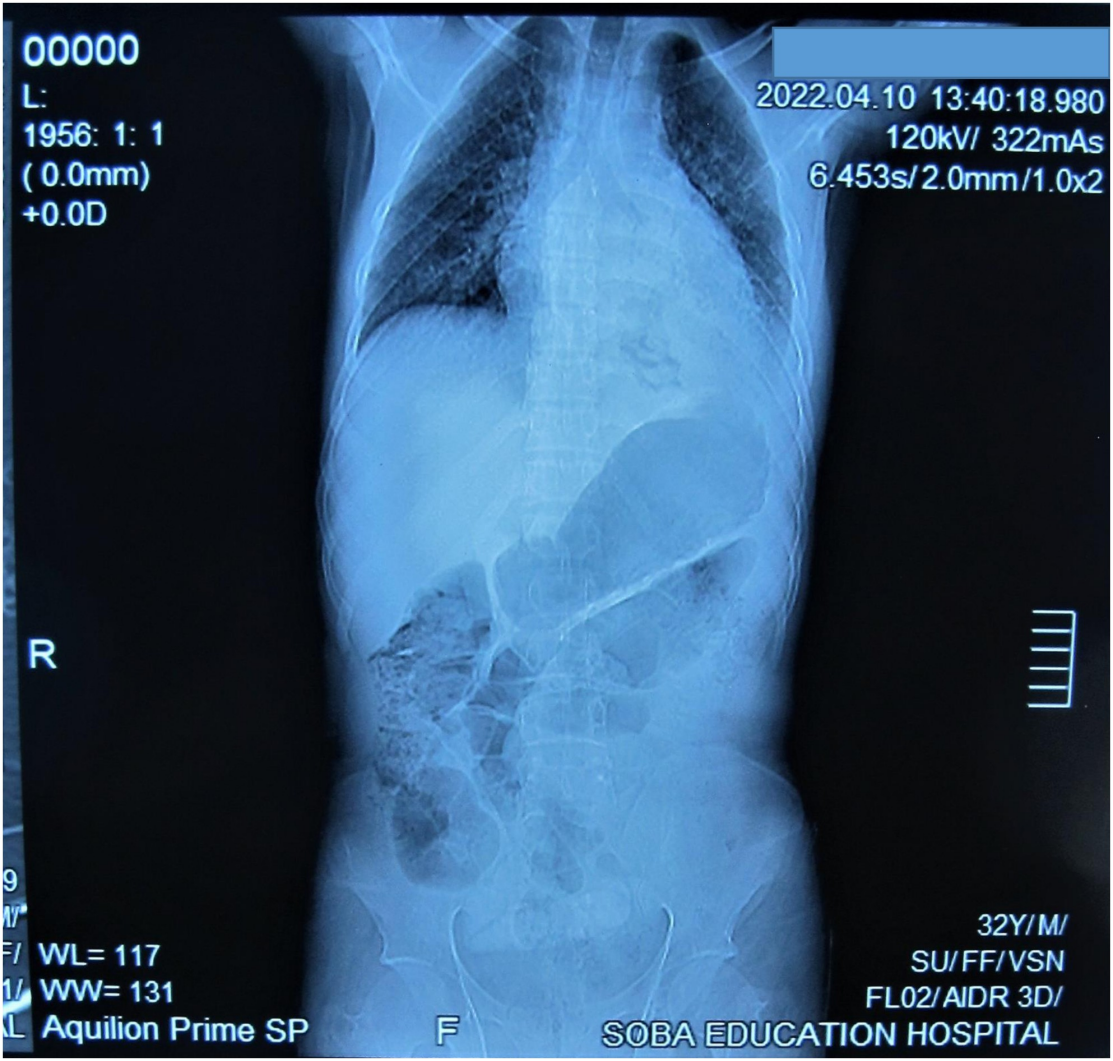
Chest CT and abdominal scan showing massive left lower lobe pulmonary consolidation and cavitary lesions.
